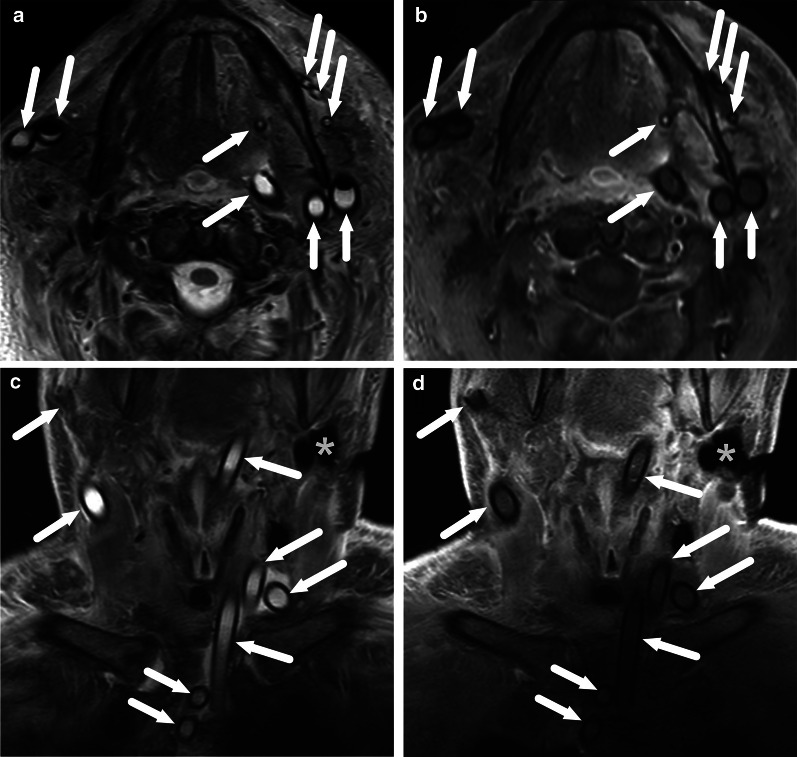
Post-treatment images of a patient with a severe neck infection, who had a total of 13 drainage tubes (arrows) and wounds intentionally left open (asterisk). Axial (a) and coronal (c) T2-weighted images and axial (b) and coronal (d) post-contrast T1-weighted images
